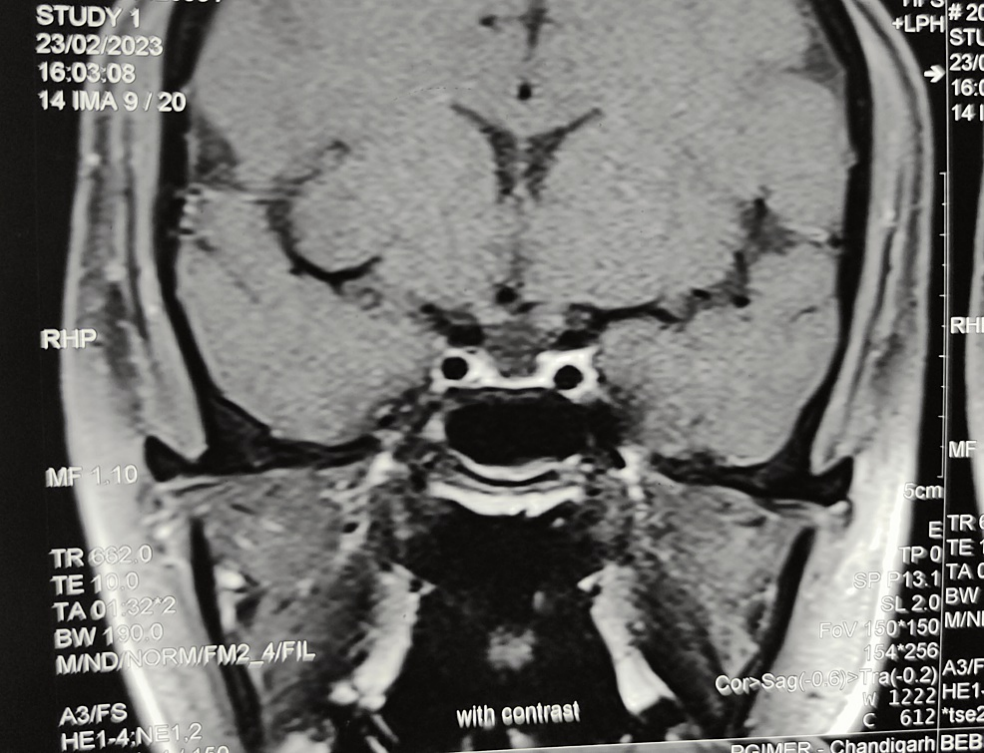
MRI showing an empty sella
